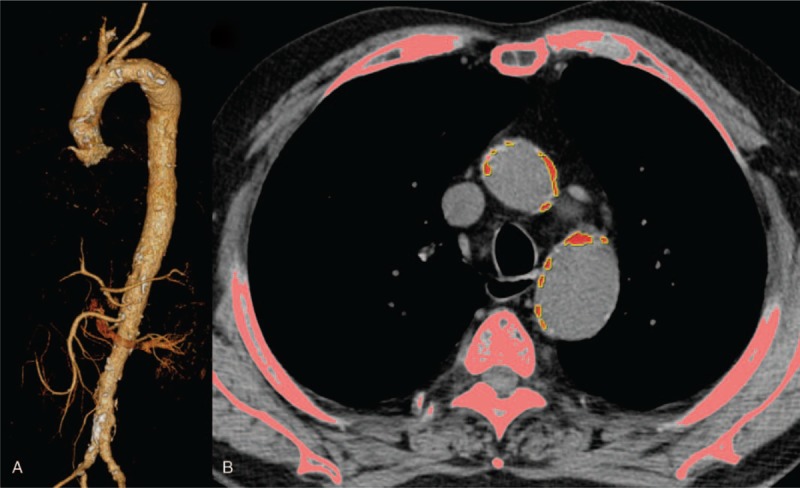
Measurement of aortic calcifications. Three-dimensional volume rendering image of thoracoabdominal CT angiography shows multiple atherosclerotic wall calcifications in the aorta, which are depicted as white patches. (A) Post-processing in the dedicated work stations enables the automated detection of bone and aortic calcifications. Semi-automated identification of aortic calcification was necessary for defining the aortic calcifications to differentiate the aortic calcium from the bone or calcified lymph nodes. Axial image obtained at the level of aortopulmonary window shows the red-colored aortic calcifications. (B) The total amount of aortic calcification measured 30,539.
